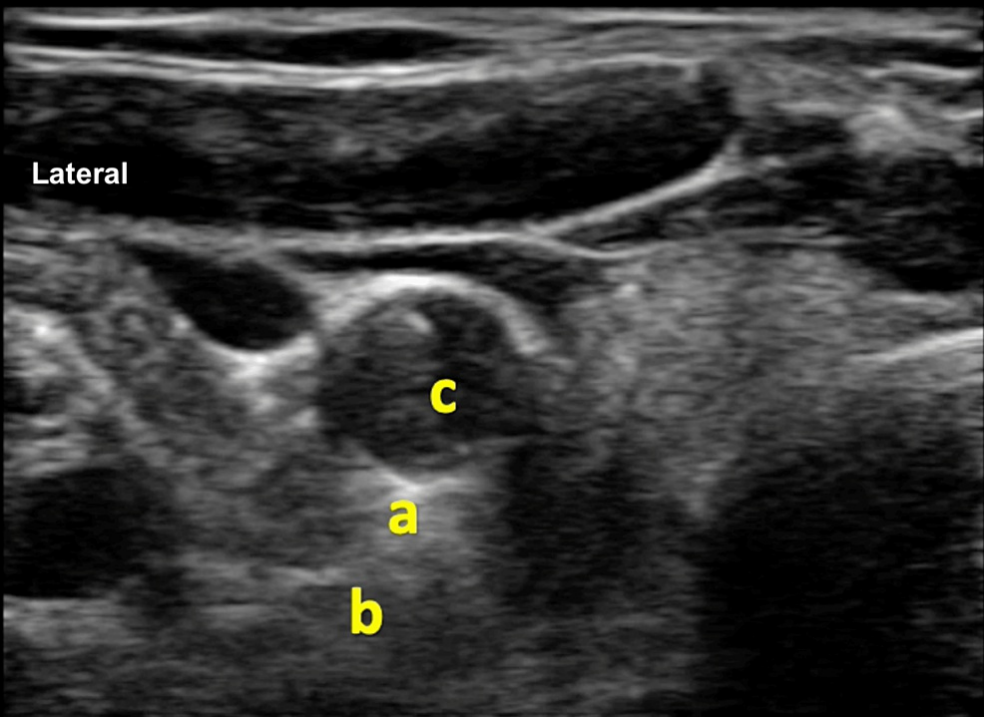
Ultrasonographic image of the right side of the neck depicting stellate ganglion a: stellate ganglion above the longus colli muscle. Site for deposition of local anesthetic solution. b: longus colli muscle. c: right carotid artery
